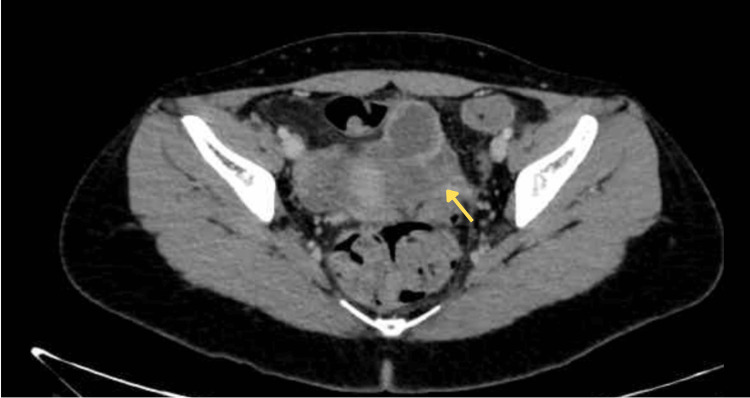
Follow-up contrast-enhanced axial CT scan obtained after initiation of anti-tuberculous therapy showing interval reduction in the size of the left adnexal lesion, consistent with therapeutic response.
